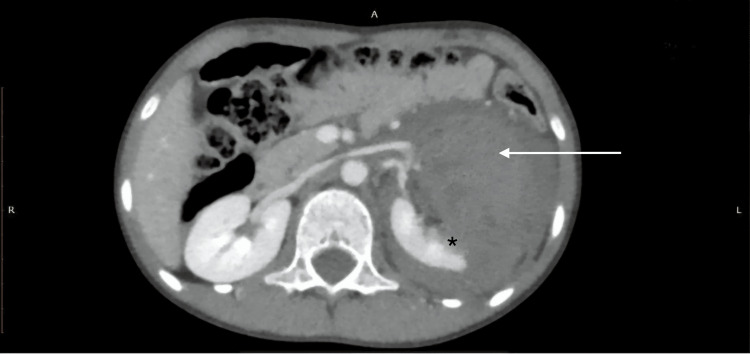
AAST grade IV left renal injury with mid-pole laceration and large perinephric hematoma on CECT (PV protocol). A nine-year-old male child with a history of RTA underwent CECT of the abdomen using the PV phase. The axial CECT image shows a left renal laceration predominantly involving the mid-pole (black star), extending to the renal hilum, with an associated large perinephric hematoma (white arrow). AAST: American Association for the Surgery of Trauma; CECT: contrast-enhanced computed tomography; PV: portal venous; RTA: road traffic accident.
